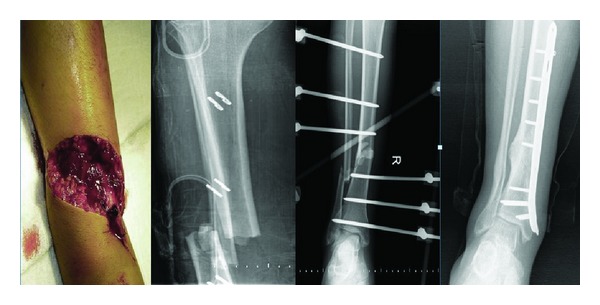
Case 13: AO/OTA: 42B2, GA: 3A, 28-year-old male, traffic accident, elective surgery (third stage): plate-screw, 14-month followup, X-ray: full union.
